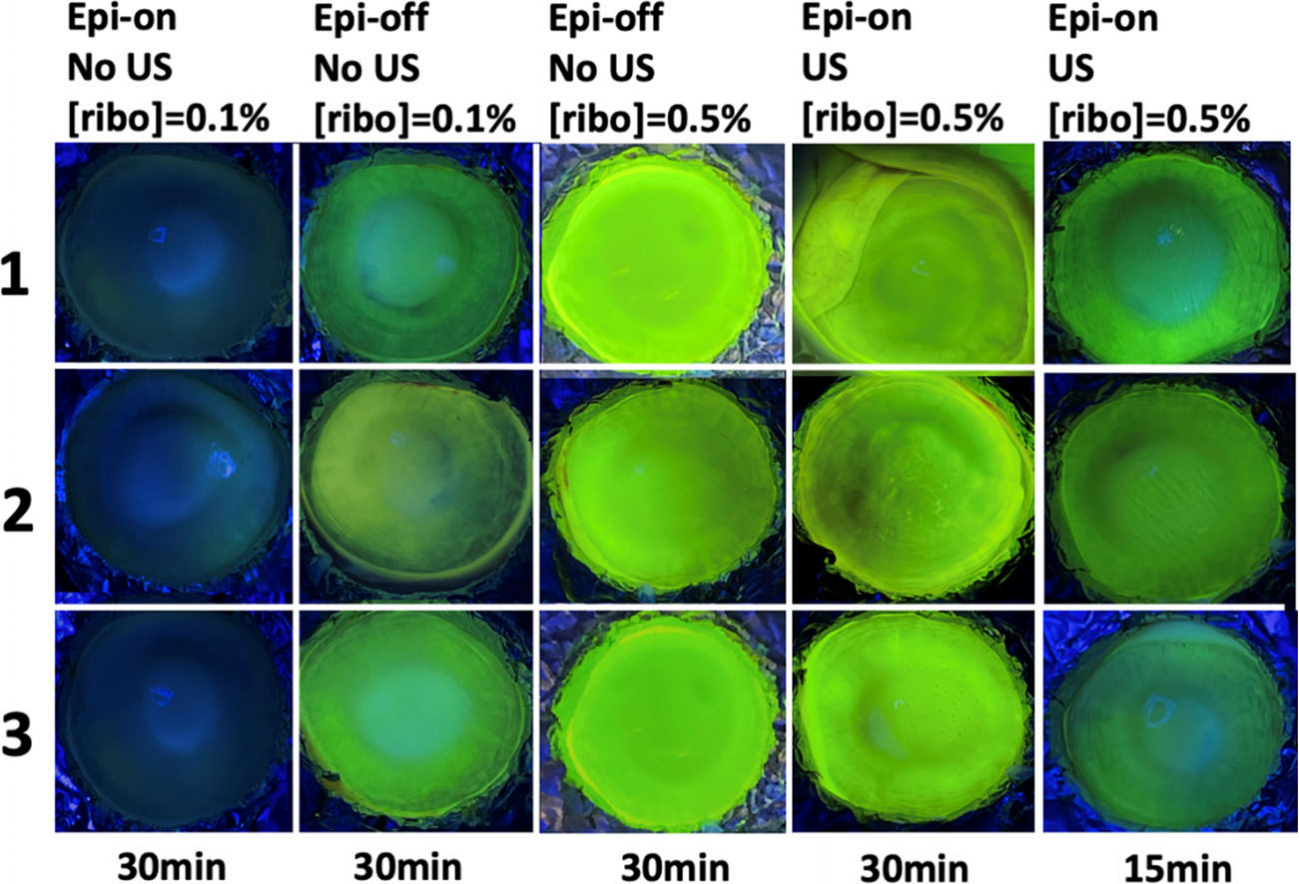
Visual images of cornea surface under UVA for qualitative assessment of riboflavin absorption from in vivo experiments. Epi‐On denote eyes with intact corneal epithelium while epi‐off denote eyes with corneal epithelium removed. Eyes were exposed to riboflavin with the indicated concentration for the duration as designated. Eyes treated with ultrasound (US) received continuous ultrasound at 40 kHz and MI = 0.8 on the intact cornea during the application of riboflavin solution. Representative images from three eyes are shown for each condition tested.
